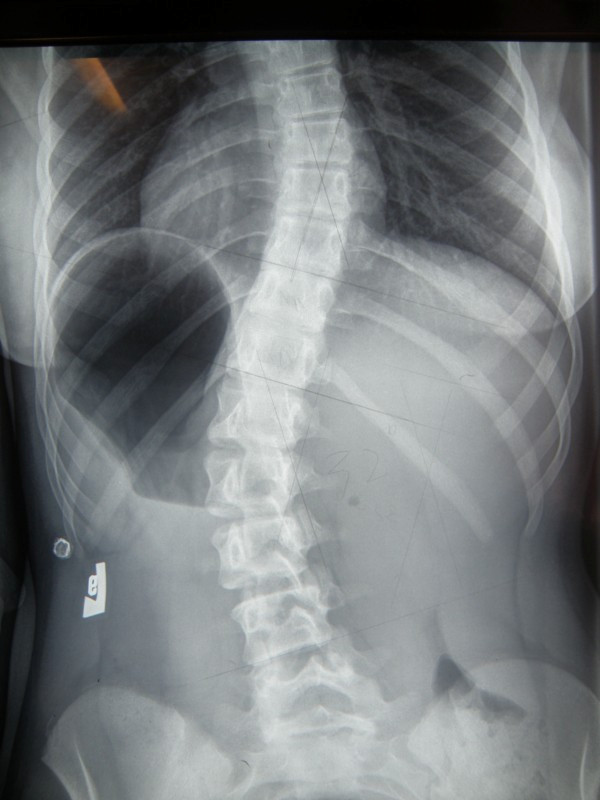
Radiography before treatment.
